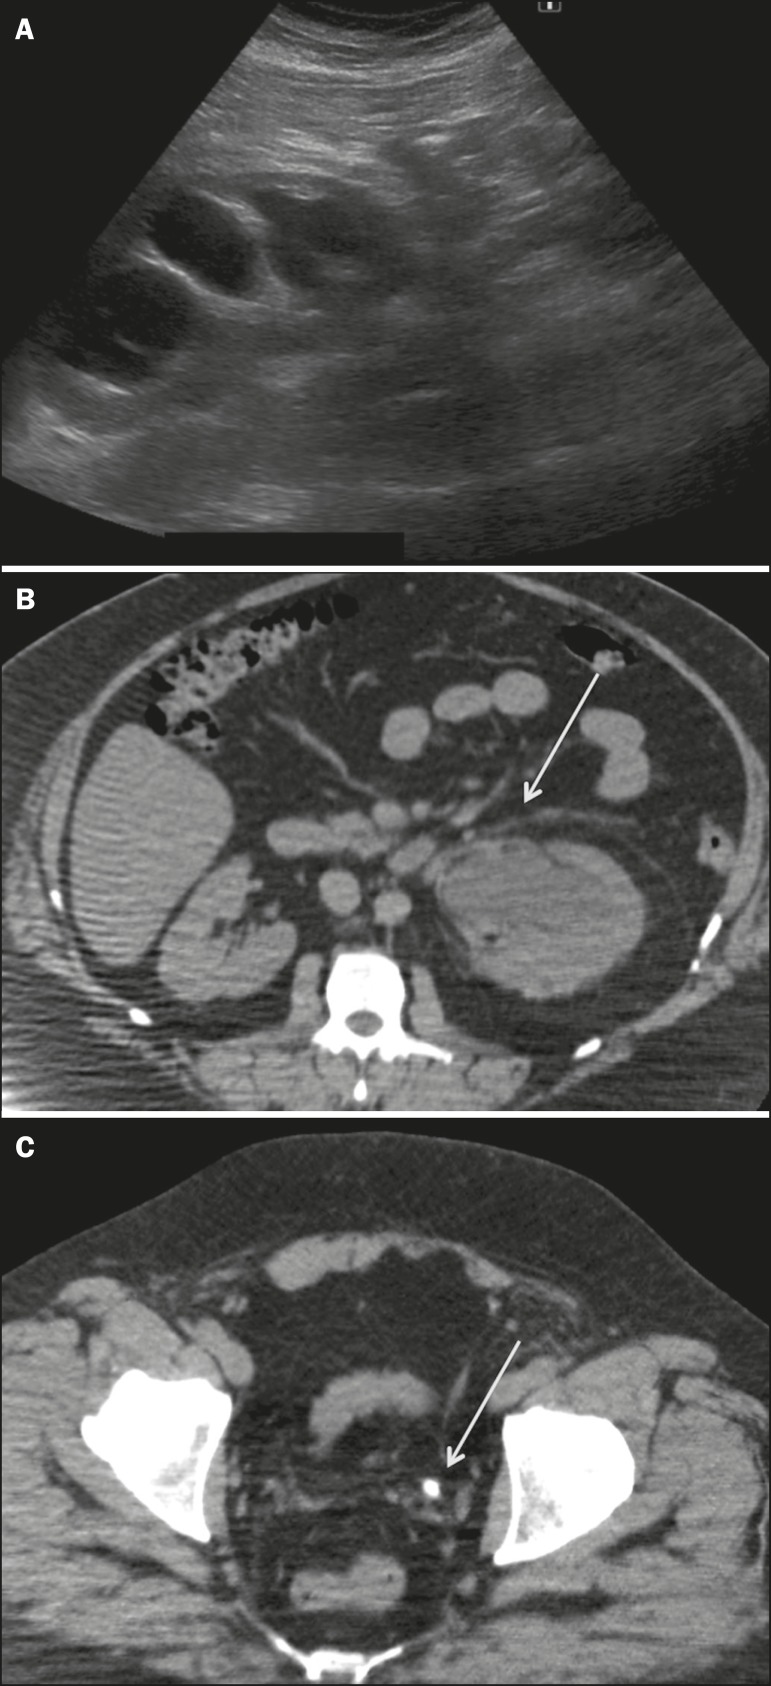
A 43-year-old male, BMI = 39, with abdominal pain. Ultrasound image
